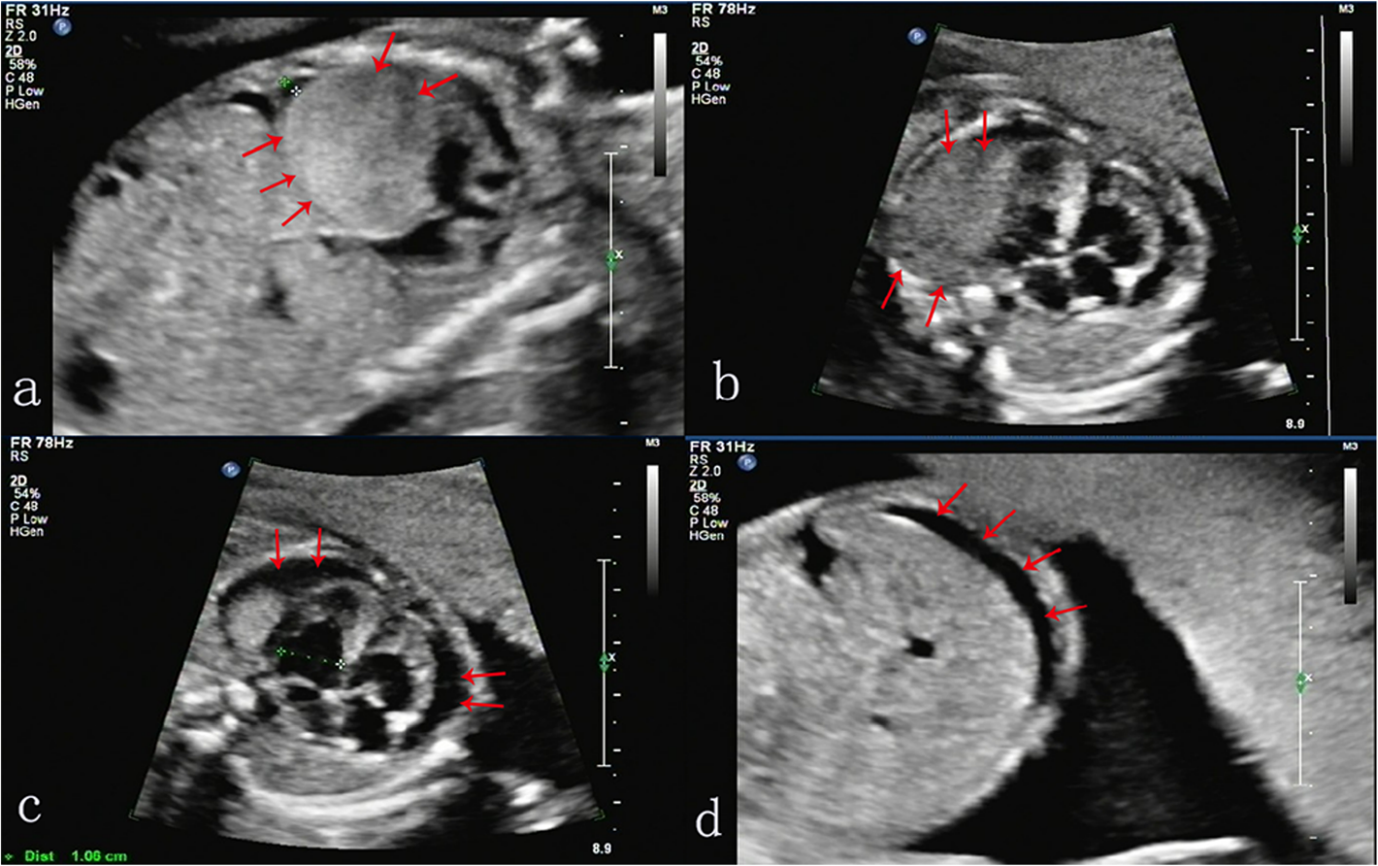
The results of fetal ultrasound showed (a, b) cardiac rhabdomyosarcoma, (c) pericardial effusion, and (d) ascites
